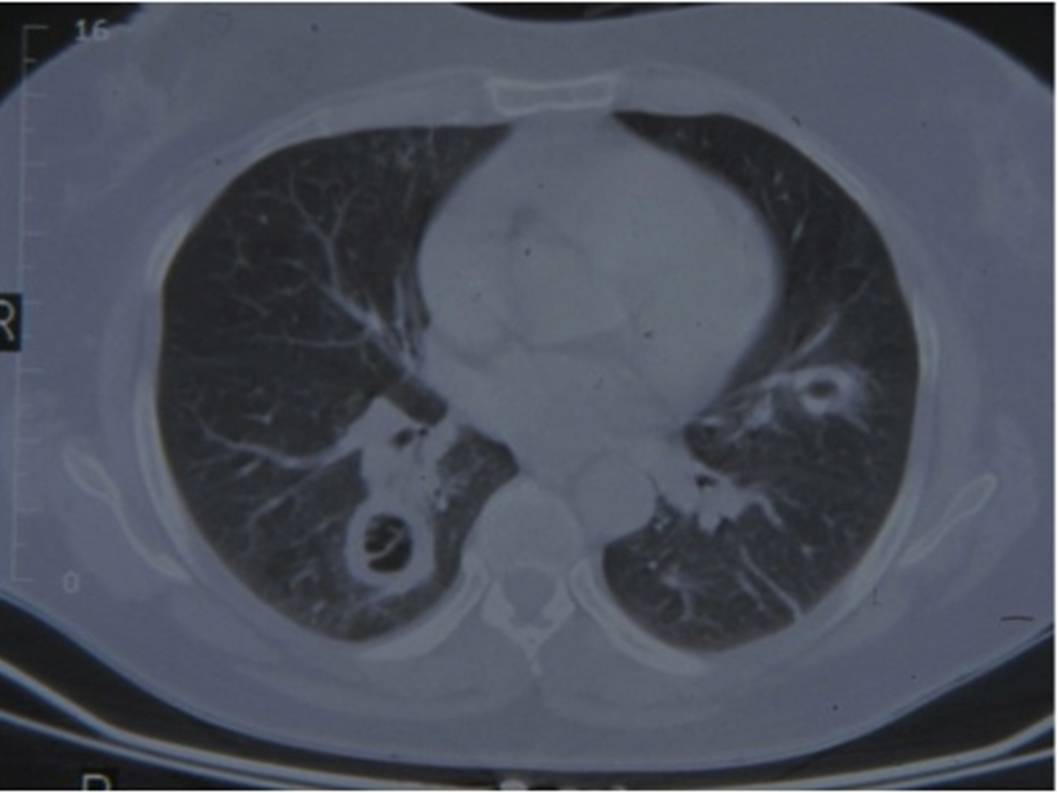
Transverse computed tomography scan shows circular masses in pulmonary fields.
